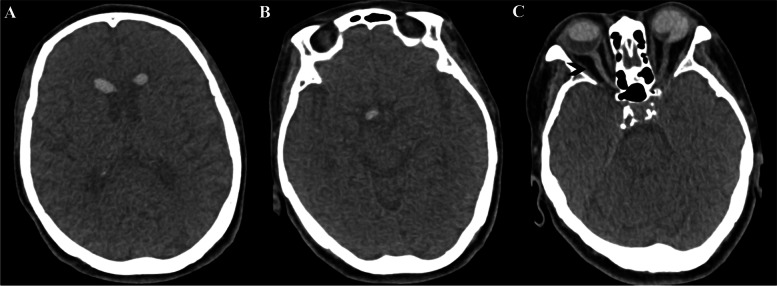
Final craniocervical CTA revealed that the silicone particles in the posterior horn had moved back to the anterior horn of the right lateral ventricle, as shown on nonenhanced brain CT, and demonstrated no enhancement of these silicone particles (A-B). The right optic nerve had a larger density than the left, which was close to intraocular silicone oil (C, black arrow)
